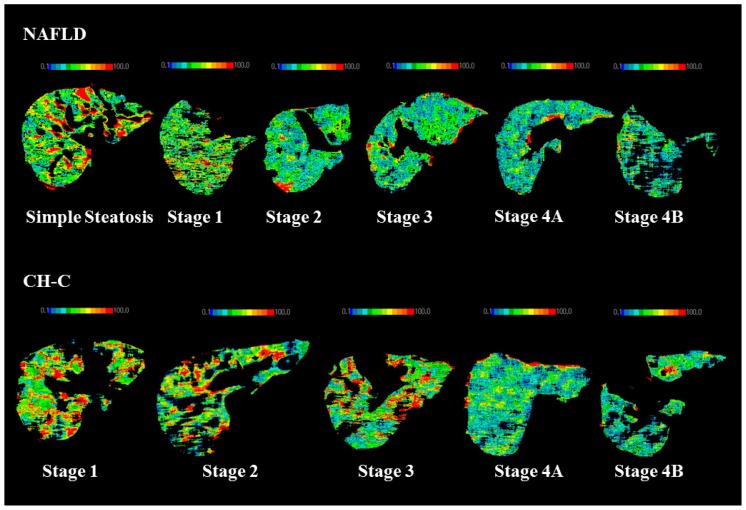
Typical PVTBF maps of NAFLD and CH-C in each fibrosis stage. Measurement of hepatic tissue blood flows (TBFs) and confidence values obtained using xenon computed tomography (Xe-CT). The color in each blood flow map changes from blue to red with increasing blood flow.
